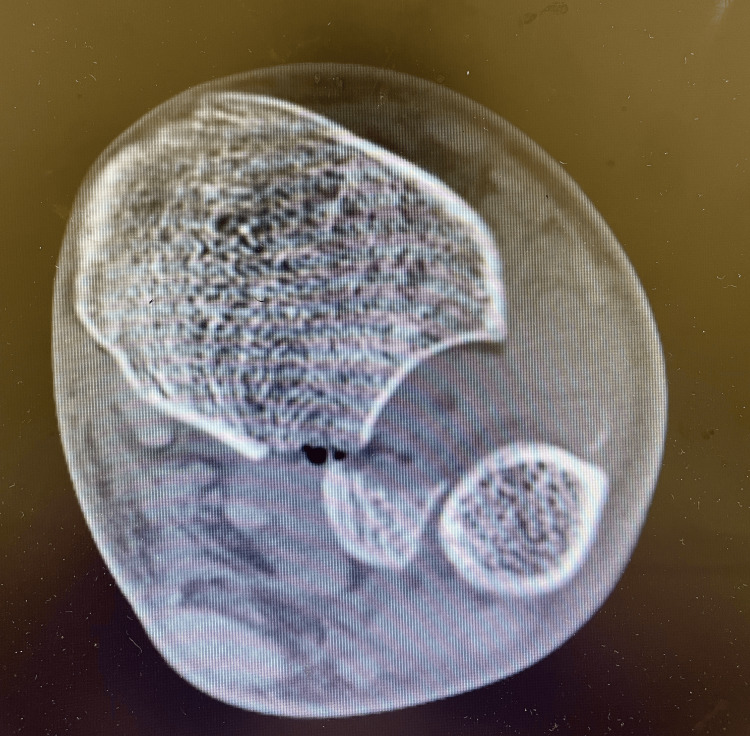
Axial CT scan of the ankle shows the posterior malleolar fracture and rupture of the anterior ligament syndesmosis.
